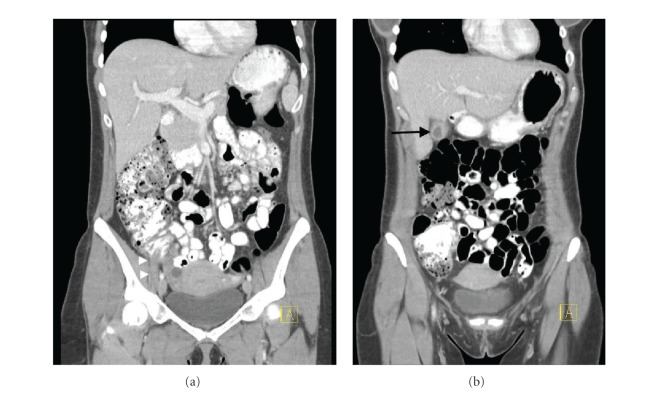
CT abdomen and pelvis, with oral and IV contrast. Note the dilated appendix (white triangles) on the left panel and the inflamed gallbladder (black arrow) on the right panel.
